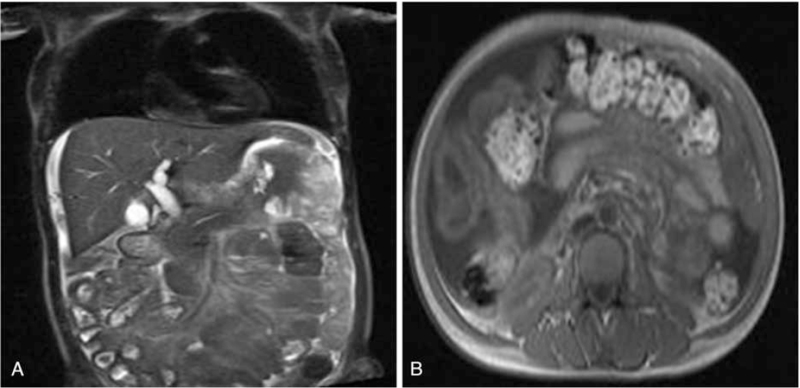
Whole abdomen MRI images showing extensive peritoneal thickening, multiple bowel-wall thickening, and edema in upper and middle abdomen.
